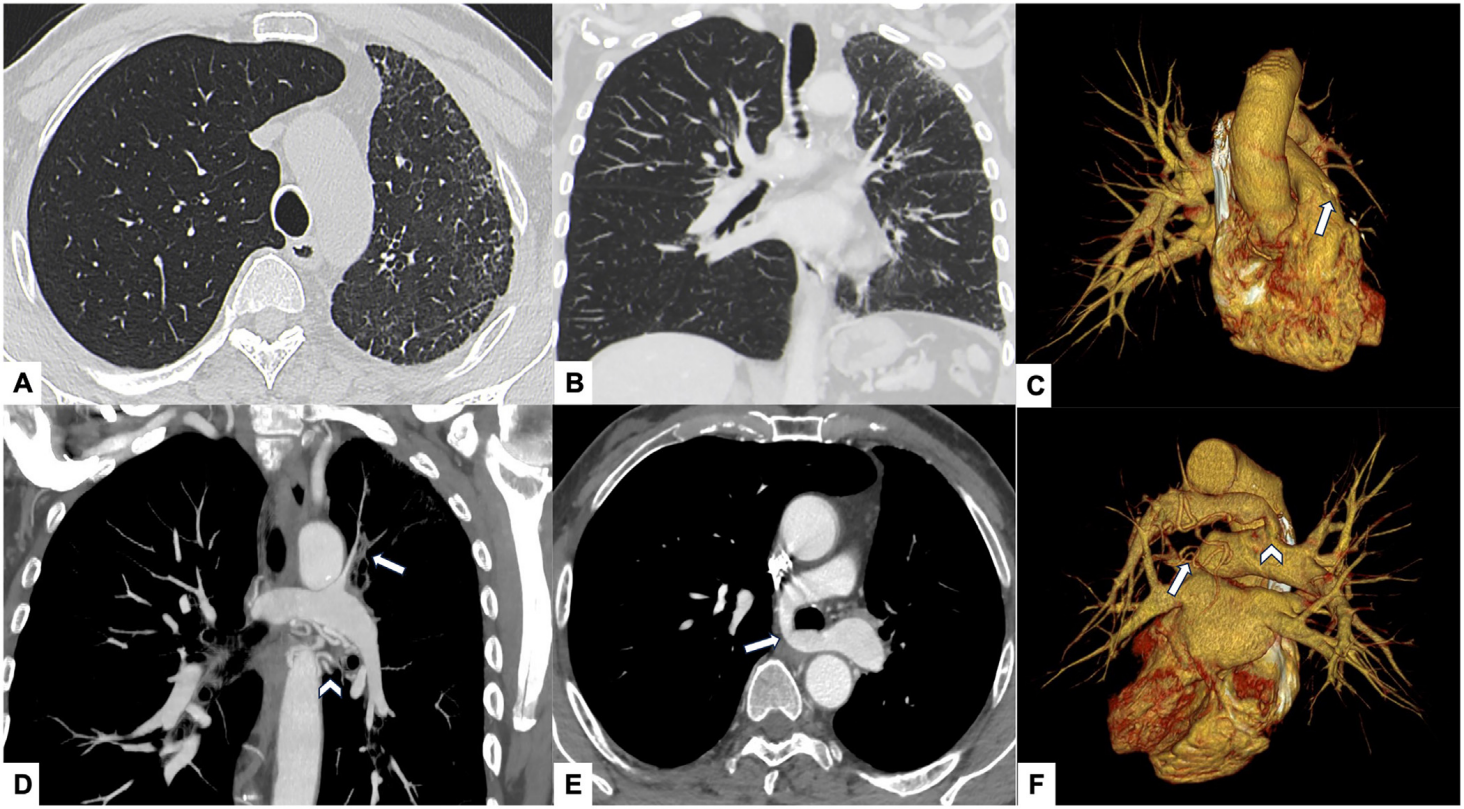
Case 3 – Pulmonary artery sling with hypoplasia of left pulmonary arterial branches and unilateral fibrotic remodeling.* Axial CT images show hypoinflation of the left lung with fine subpleural interstitial thickening (A, B). After contrast administration, an aberrant left pulmonary artery originating from the right pulmonary artery and coursing retrotracheally is demonstrated (arrow in C), consistent with a pulmonary artery sling. Hypoplasia of several left pulmonary arterial branches (arrow in D) along with hypertrophy of bronchial collateral arteries (arrowhead in D) are also evident. VRT 3D reconstruction (E, F) confirms the absence of the left pulmonary artery in its expected anatomic position (arrow in E and F) and highlights the compensatory systemic collateral circulation (arrowhead in E).
